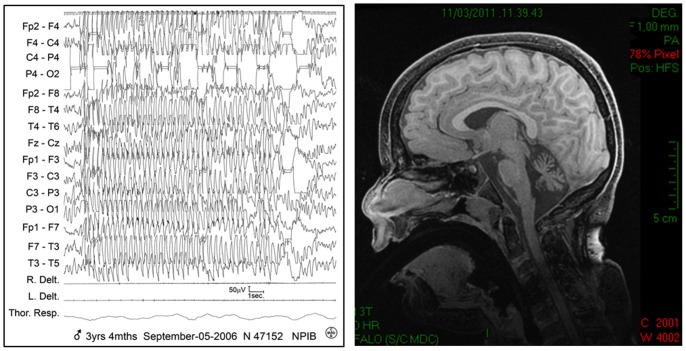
Instrumental findings in the proband. Left panel: EEG recording during wakefulness showing generalized spike-wave complex at 4 Hz lasting thirteen seconds with absence, eye up deviation and eyelid myoclonia. Right panel: Sagittal T1 MRI showing prominent cerebellar atrophy.
